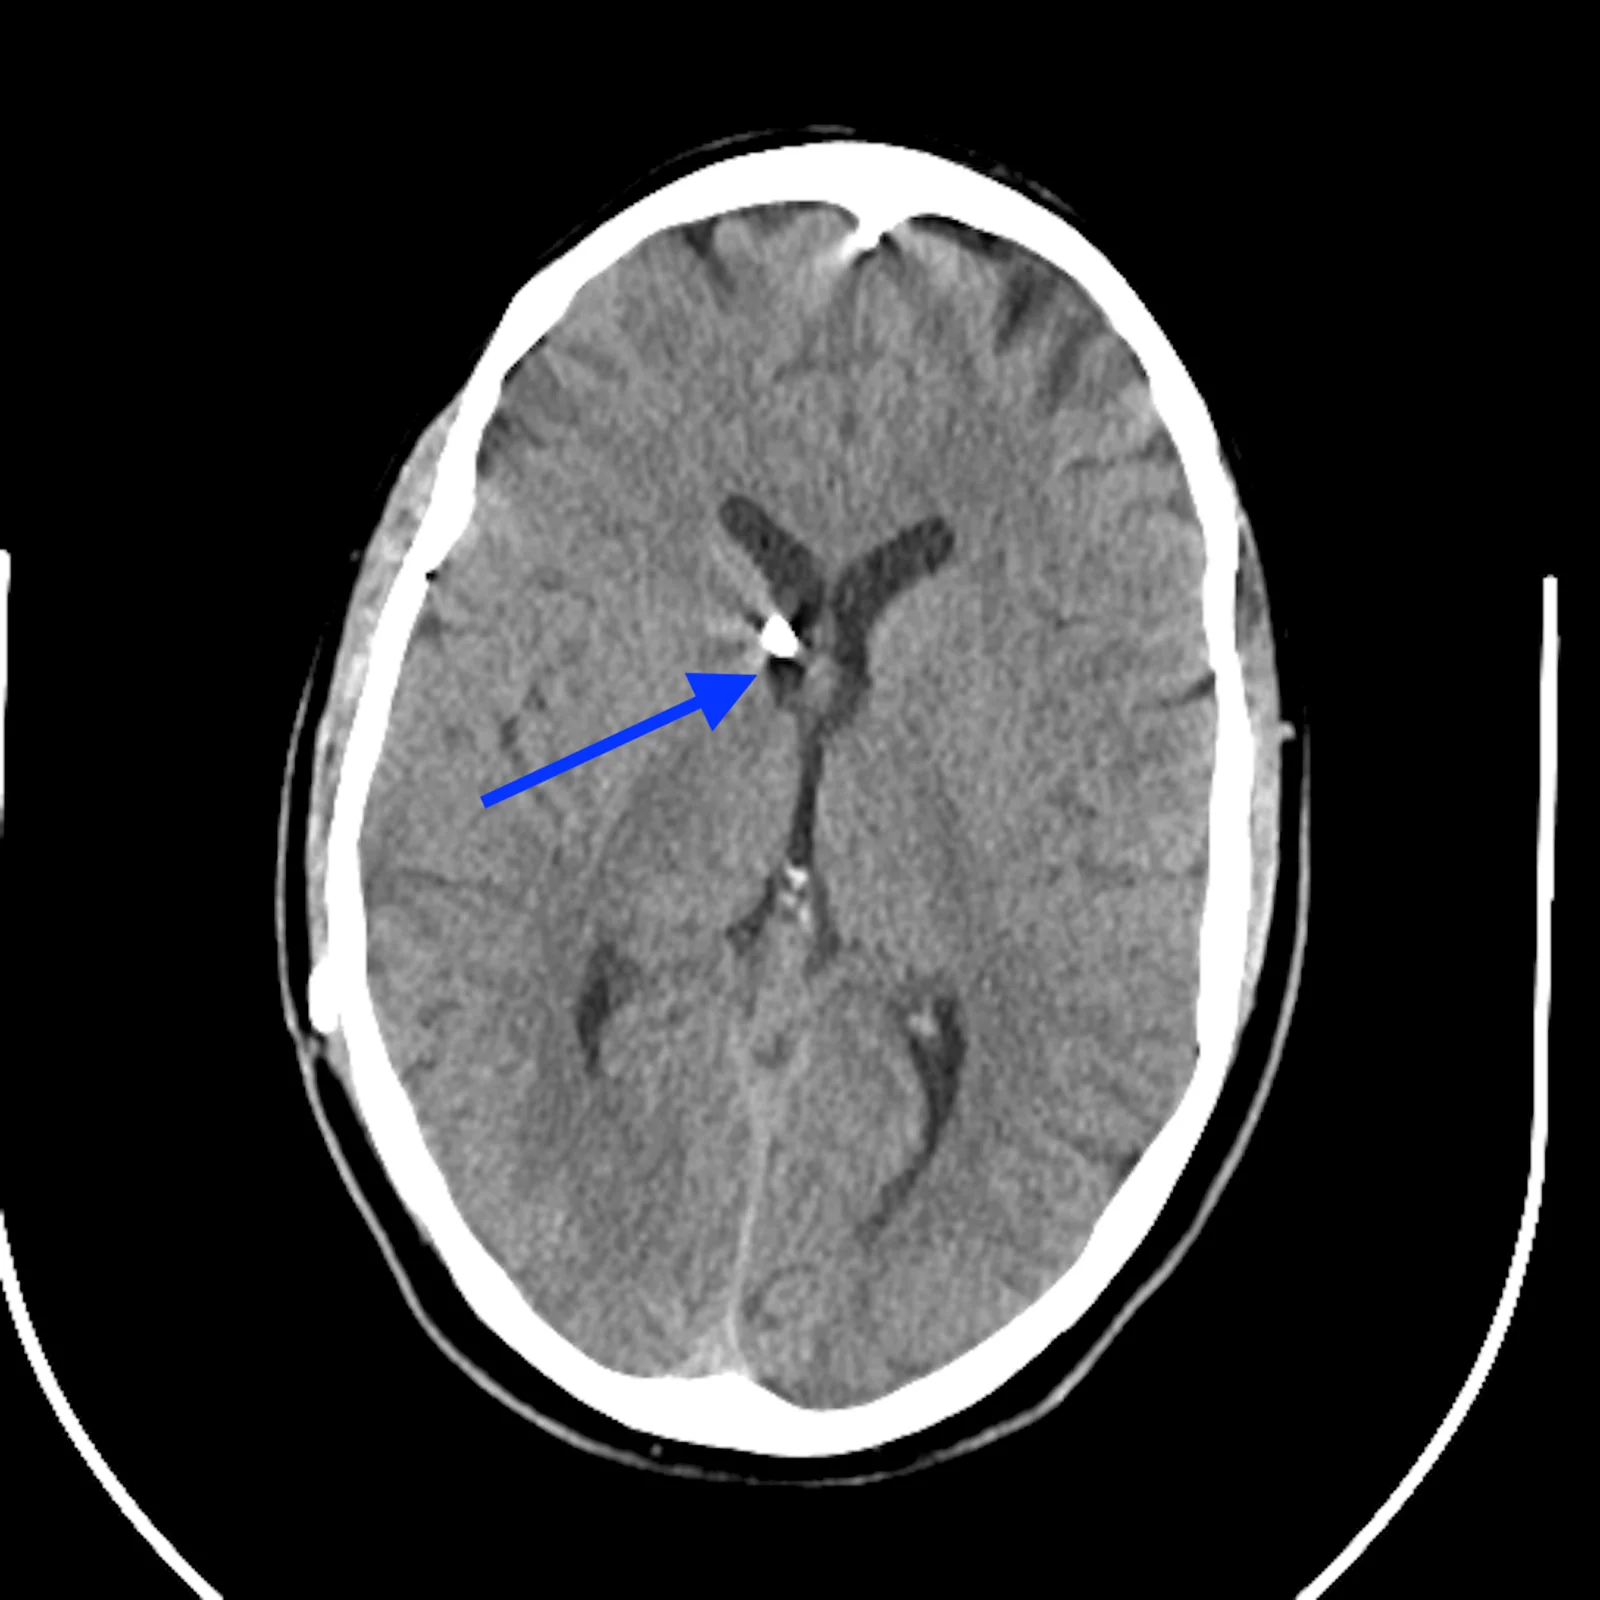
Axial non-contrast CT of the head following jugular venous compression with a soft cervical collar, demonstrating reduction in ventricular caliber: third ventricular width 4 mm, temporal horns 3.8 mm (R) and 3.8 mm (L), and Evans ratio 0.28.
